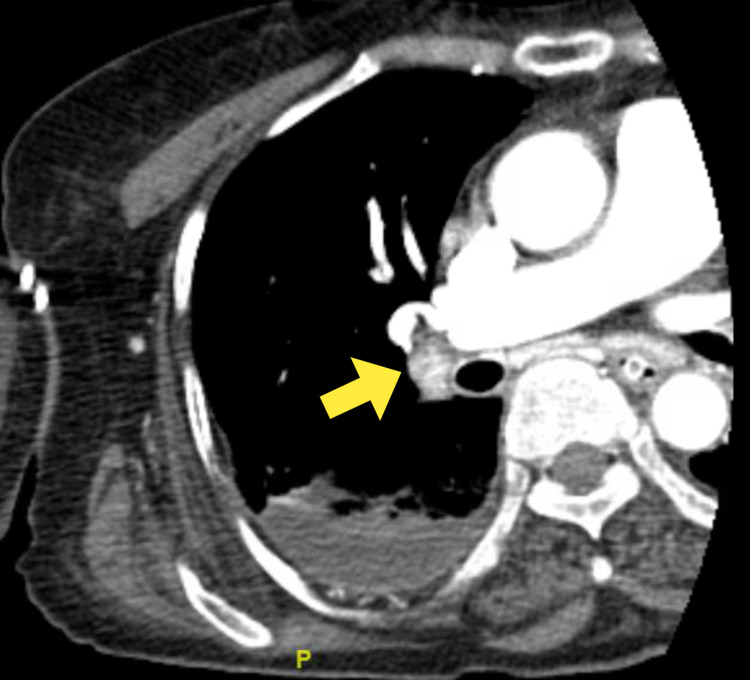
Computed tomography of the chest showing a pulmonary embolism in the right main pulmonary artery (yellow arrow)
